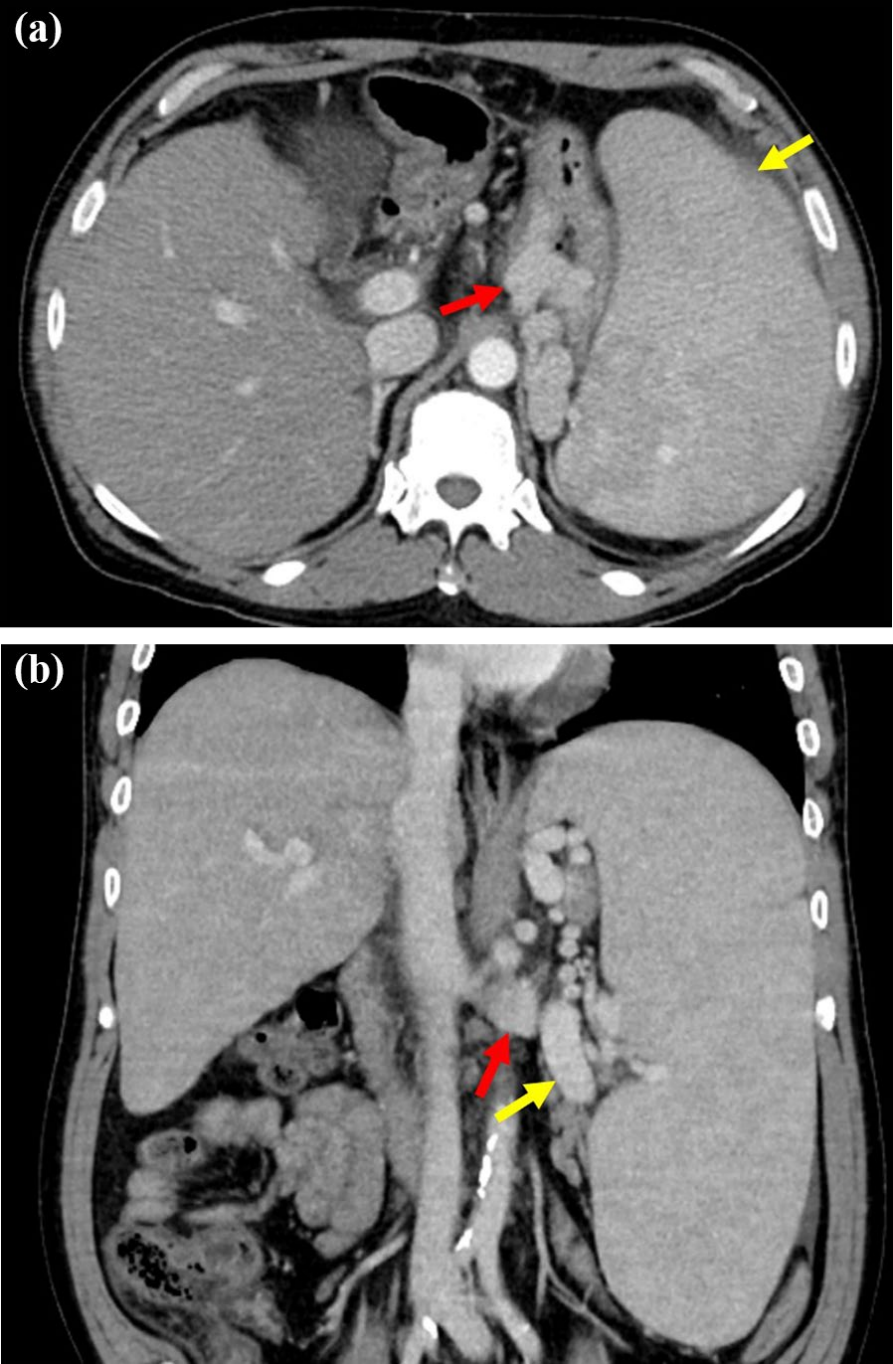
Contrast-enhanced CT before splenectomy. a An axial CT scan showing marked splenomegaly (yellow arrow) and gastric varices (red arrow). b A coronal CT scan showing an enlarged splenic vein (yellow arrow) and development of collateral vessels from the left gastric vein to a gastro-renal shunt (red arrow). *CT* computed tomography
